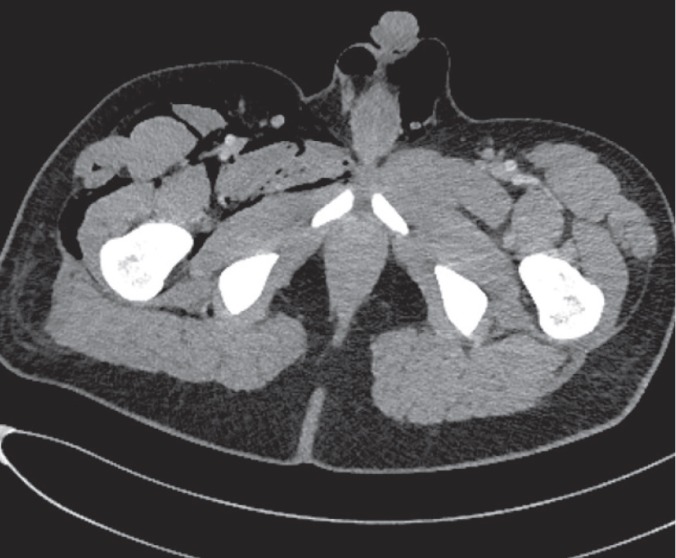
The axial CT image shows pneumoscrotum
